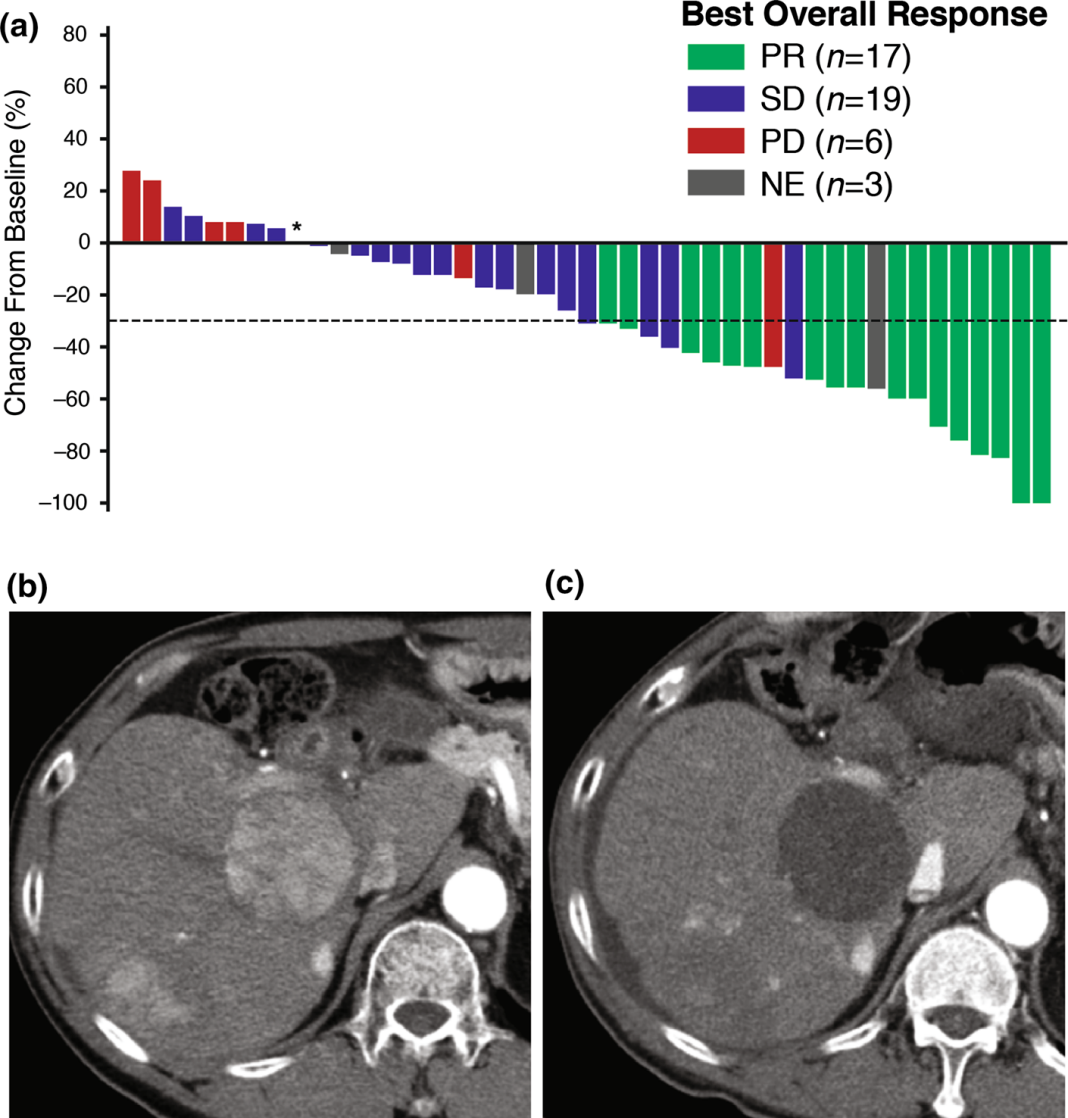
a Waterfall plot of changes in tumor size by IRRC assessment. One patient was excluded from the plot due to lack of IRRC assessment of target legion at baseline. The patient marked with an asterisk showed a best overall response of SD. b Representative liver lesion of HCC at baseline on arterial phase CT. c Representative liver lesion on arterial phase CT after 1 year of lenvatinib treatment. *CT* computed tomography, *HCC* hepatocellular carcinoma, *IRRC* independent radiologic review committee, *PD* progressive disease, *PR* partial response, *SD* stable disease, *NE* not evaluable
